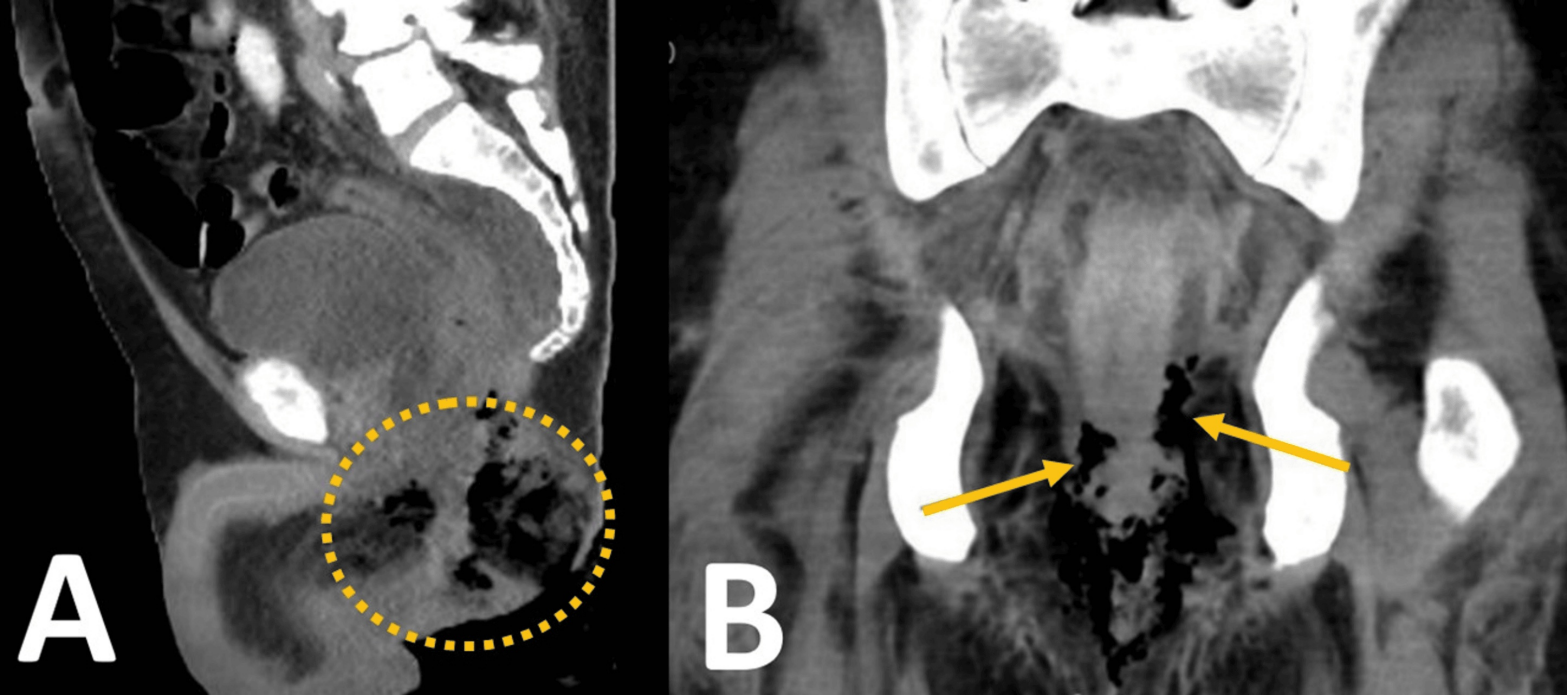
CT findings in this case of Fournier’s gangrene. (A) Sagittal view shows perirectal soft tissue gas and a large presacral fluid collection (green circle).
